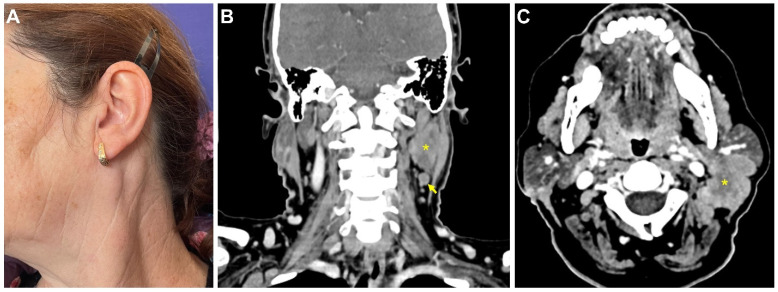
Clinical and imaging aspects at presentation. Clinical aspect (A). Contrast-enhanced CT scan of the neck in the coronal (B) and axial (C) planes. Large necrotic tumor (*) located on the posterior and inferior aspect of the left parotid gland, with infiltration of the adjacent muscles and enlarged regional lymph nodes (arrow).
